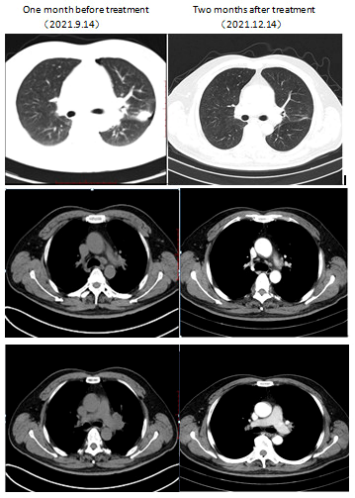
Computed tomography imaging showed initial response to combination of dabrafenib and trametinib. Left column: CT scan of the chest at baseline 1 month prior to dabrafenib plus trametinib therapy demonstrated a nodule in the upper left lung (lung window) accompanied by enlargement of multiple lymph nodes of the left lung hilum as well as mediastinum (mediastinal window). Right column: CT scan at 2 months after therapy demonstrated a marked response with a significant decrease in the nodule, left lung hilum, and mediastinum.
